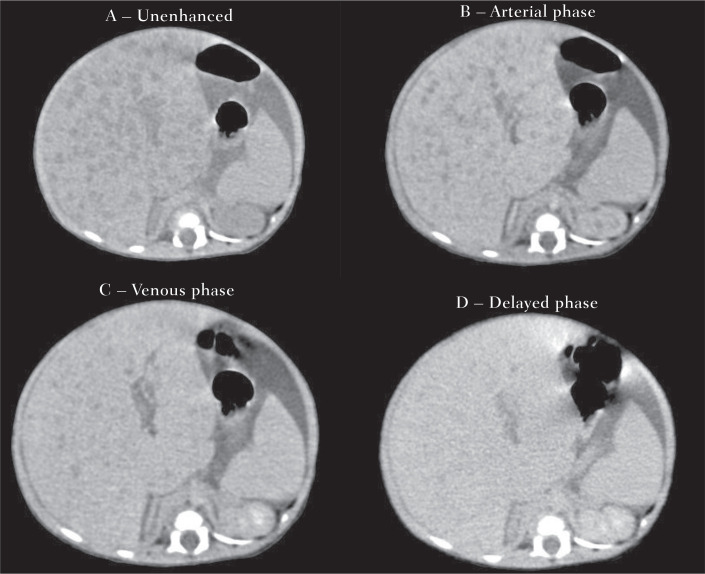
Hepatic IHs. Further CT investigation to evaluate nodules.
